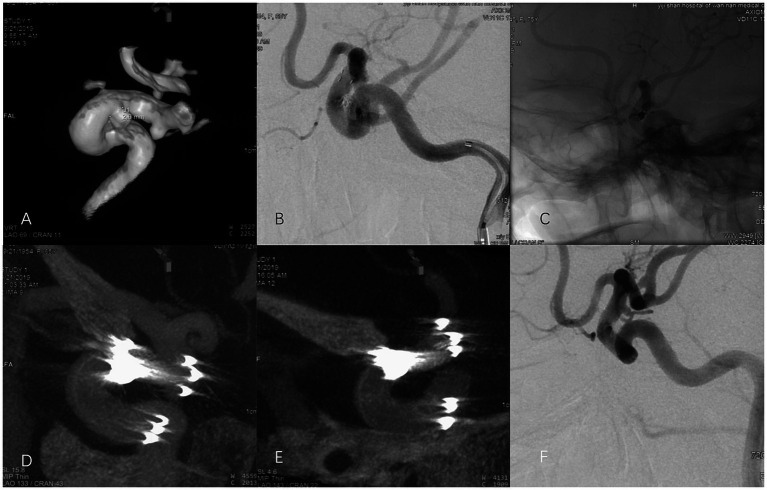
Representative images of ISA in a 62-year-old female with an unruptured right OSA treated with an EP-VRD2 stent. (A) 3D reconstruction of DSA showing the right OSA. (B, C) Immediate postoperative angiography revealed complete occlusion (RROC I), with (B) subtraction and (C) un-subtraction images. The stent size was 4.0 × 23 mm. (D) Dyna CTA showed a “CS” between the outer arc of the stent and the curved ICA siphon, with asymmetric proximal markers. (D) Dyna CTA showed a “CS” between outer arc of the EP-VRD2 and curved ICA siphon after initial deployment. The proximal markers were asymmetric and the distal markers were symmetric. (E) A small CS on the outer arc of the stent persisted on Dyna CTA even after attempts at stent massage with a micro-guidewire. (F) DSA after 17 months indicated complete occlusion.
